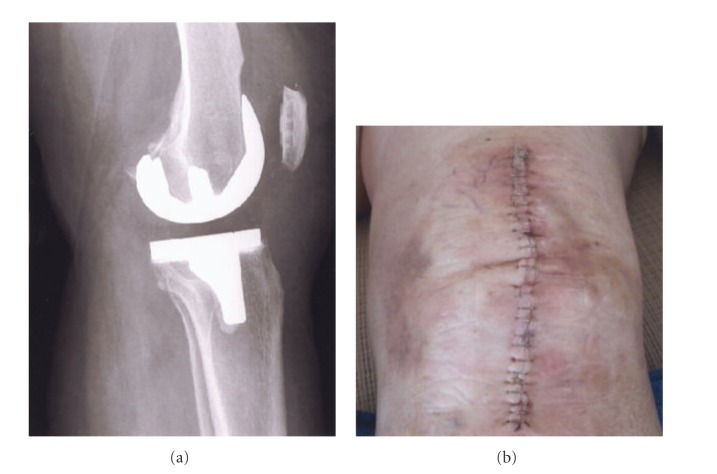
X-ray image (a) and incision-suture photograph for total knee replacement (b) (Courtesy of Patricia Murr).
